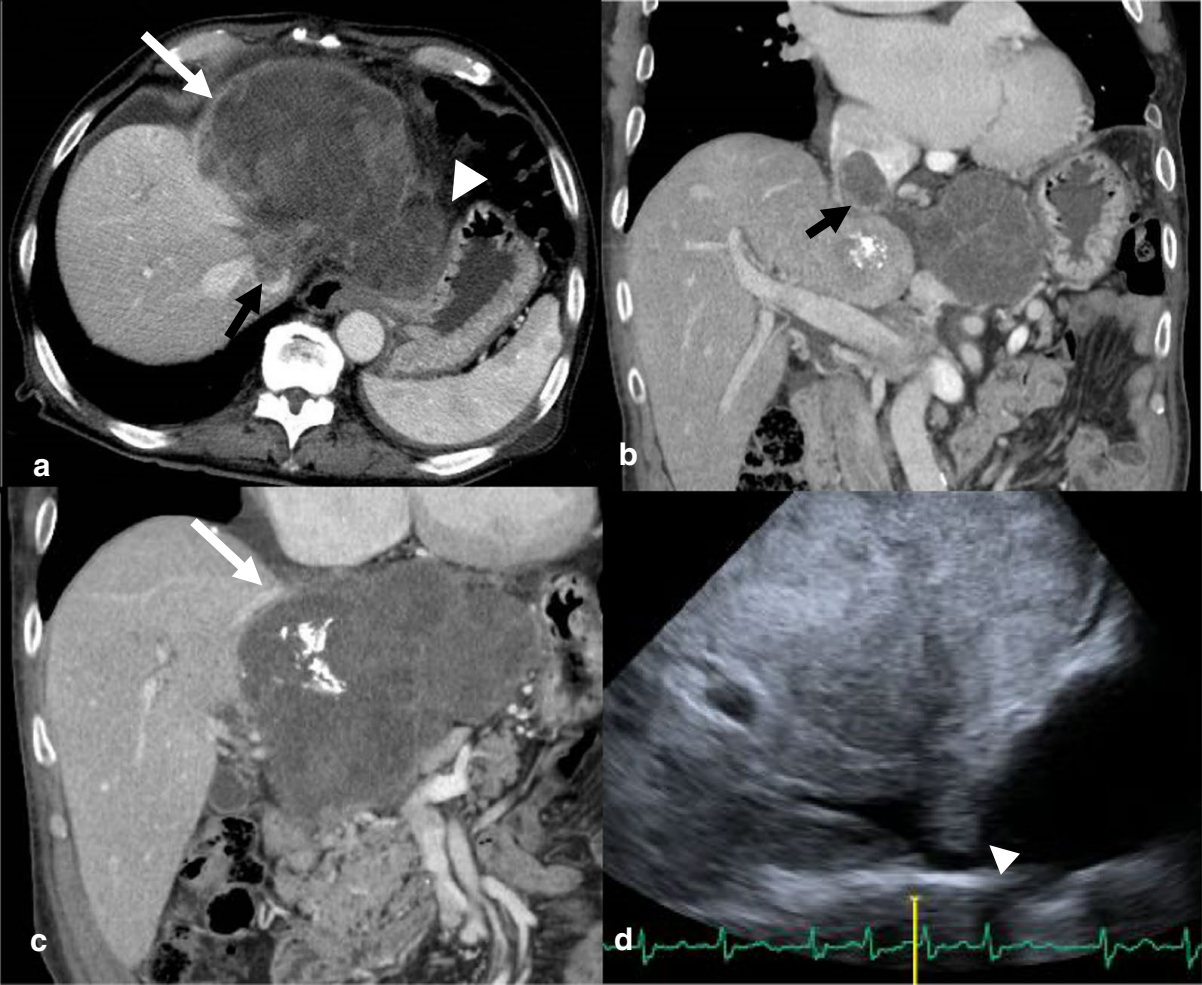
Diagnostic imaging. a Enhanced computed tomography scan showing a 13-cm tumor in the left lobe of the liver (long white arrow), with a tumor thrombus arising from the left hepatic vein into the inferior vena cava (short black arrow), and enlarged lymph nodes (white arrow head). b Coronal section image showing the tumor thrombus extending from the left hepatic vein via the inferior vena cava to the right atrium (short black arrow). c The main tumor was seemingly attached to the middle hepatic vein, but did not involve it (white arrow). d Transthoracic echocardiography showing a tumor thrombus extending from the inferior vena cava to the right atrium (short white arrow)
